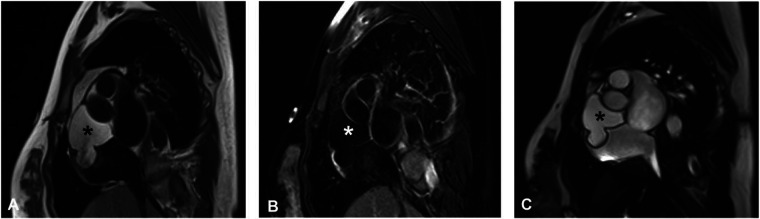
Characterization of the lipoma by CMR. (A) T1-weighted imaging demonstrates a well-circumscribed hyperintense mass (*) in the right pericardial region. (B) Fat-suppressed (STIR) sequence shows complete signal attenuation of the lesion (*). (C) Post-contrast T1-weighted imaging shows no enhancement of the mass (*).
